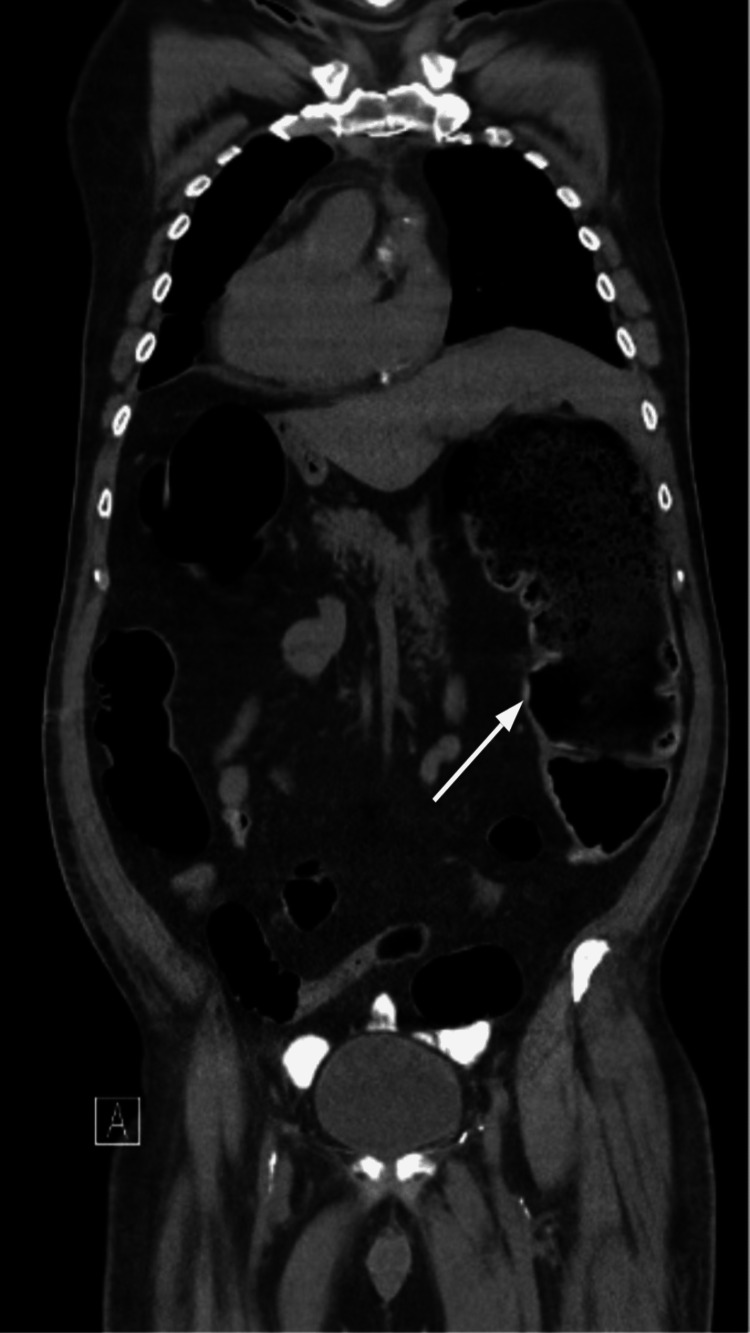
Abdominal CT - coronal view The arrow demonstrates cecum distention of around 9 cm CT: computed tomography
